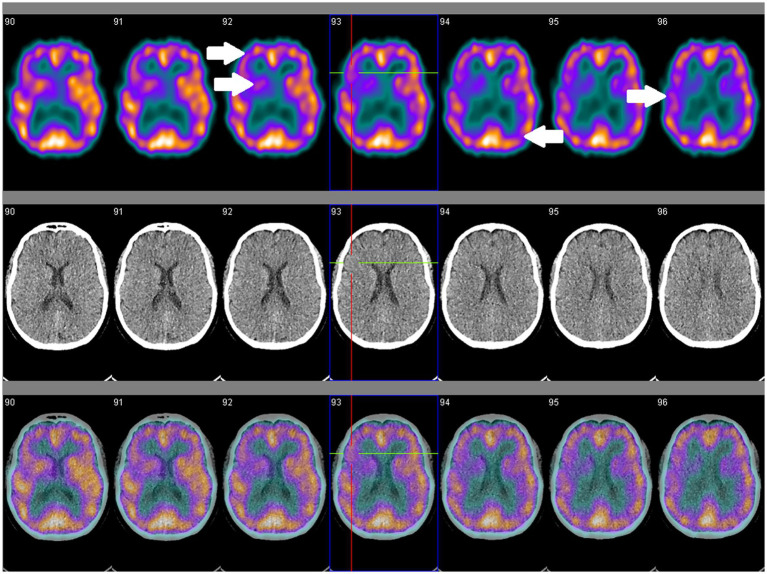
SPECT coregistered to CT (SPECT/CT) in a 56-year old female with clinically and serologically inactive SLE and no overt neuropsychiatric symptoms showing asymmetrical perfusion. Comparison between right and left sides are necessary for qualitative assessment. Focal areas of hypoperfusion (arrows) are seen in the frontal, temporal and occipital lobes, and the caudate, putamen and thalamus.
